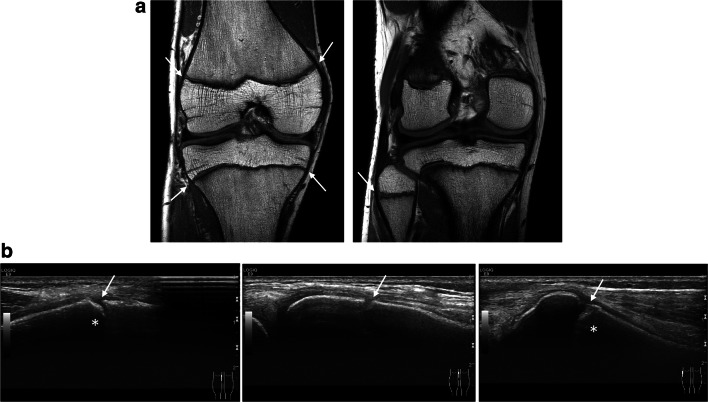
Right knee of a 15-year-old boy showing wide open physeal plates (arrows) on a coronal T1-weighted MRI (a) and longitudinally oriented US images (b) on the medial distal femur (left image), the medial proximal tibia (middle image), and the proximal fibula (right image). On the US, note the gap between the metaphyseal and epiphyseal cortex and the linear, echogenic zone of calcification continuing transversally into the physis (asterisk, US-S1)
